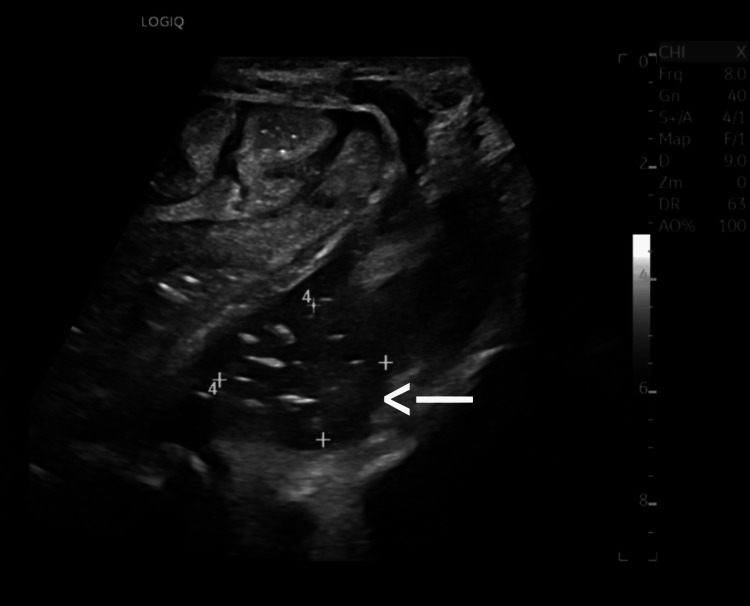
Ultrasonographic appearance of localized inflammatory changes in the proximal jejunal mesentery suggestive of early abscess formation (white arrow).
